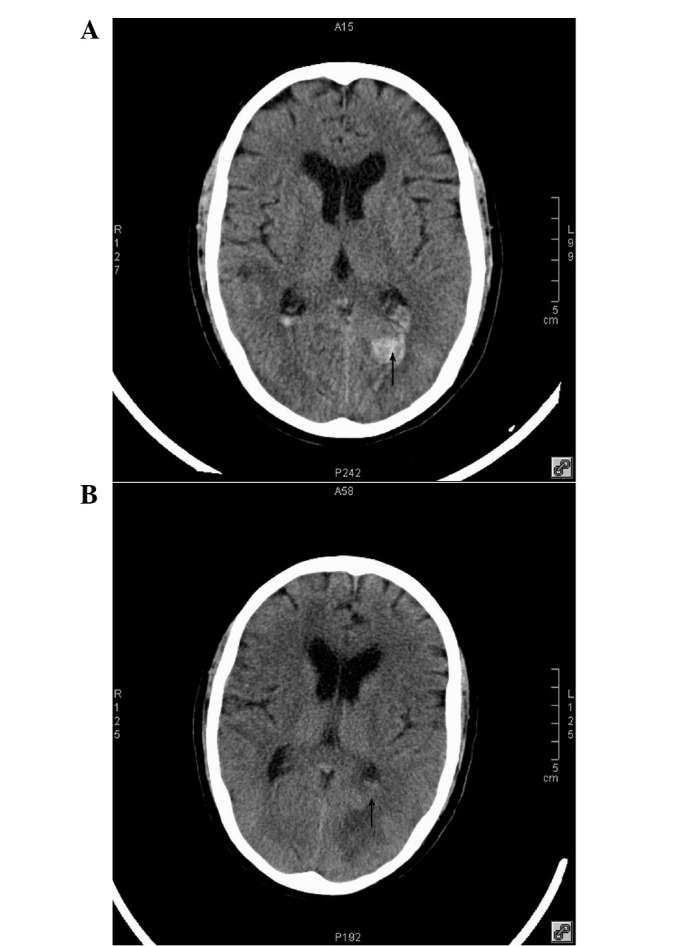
Cranial computed tomography film of Case 2. (A) Multiple regional high-density areas were observed following intracranial hemorrhage. (B) Improvement in hemorrhage following treatment. The arrows in the images indicate the place of bleeding.
